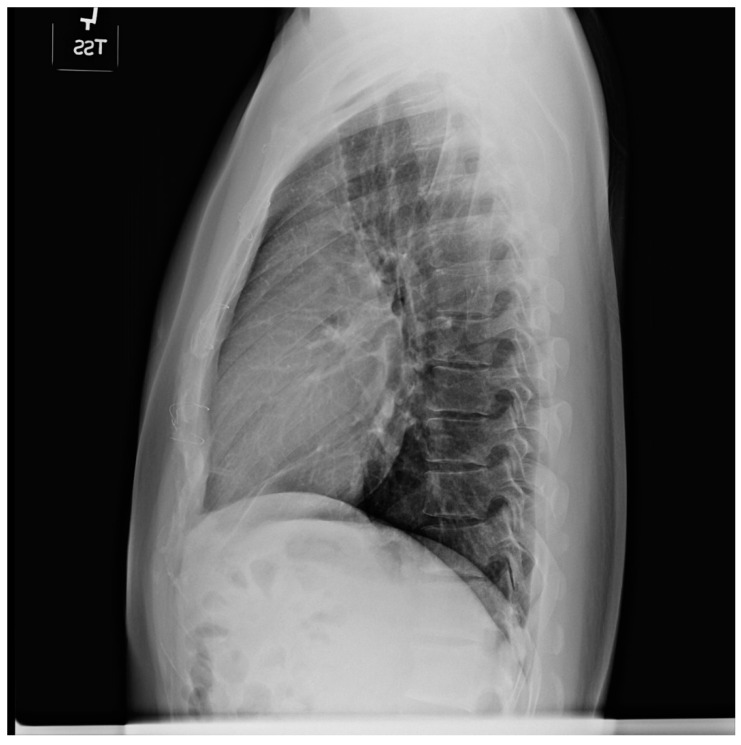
Lateral chest X-ray in congenitally corrected transposition of the great arteries in adulthood: this is the lateral chest X-ray of the same 41-year-old male in Figure 13. There are sternal wires from the median sternotomy used to repair his atrial septal defect, remote from this presentation. The retrosternal airspace is opacified, suggesting right ventricular enlargement. On examination he had a holosystolic murmur of atrioventricular valve regurgitation. Doppler echocardiography demonstrated severe systemic atrioventricular valve regurgitation (the anatomic tricuspid valve), and moderate dilatation of the systemic ventricle (the anatomic right ventricle) with preserved systolic function. He underwent replacement of the systemic atrioventricular valve with a dual-tilting disc mechanical prosthesis and did well. Optimally, the transition from pediatric congenital heart disease care to adult congenital heart disease care should be a coordinated hand-off [12].
